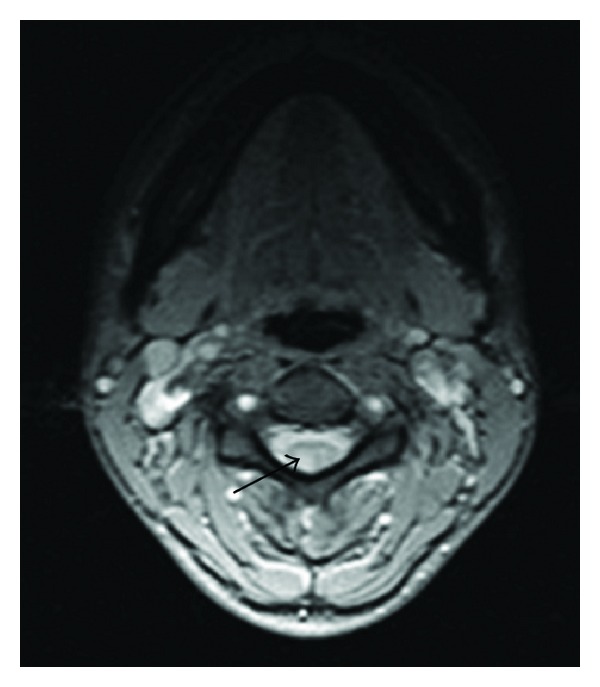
MRI 1.5T Axial section obtained with sequence GRE-T2 at C4 level showing a signal hyperintensity of posterior columns.
